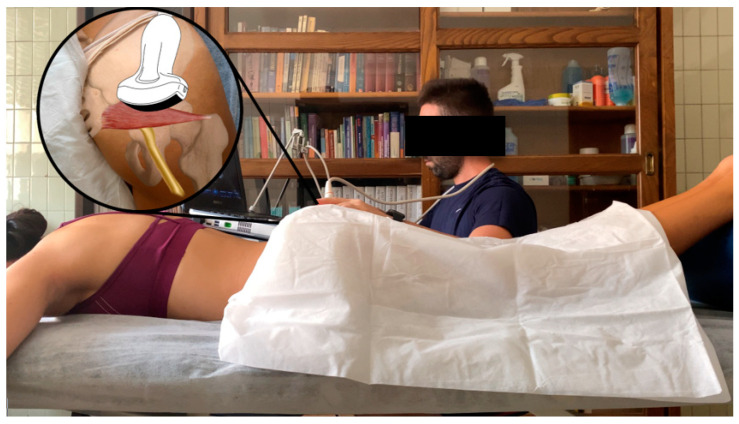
Ultrasound imaging acquisition: participants’ position and probe positioning.
